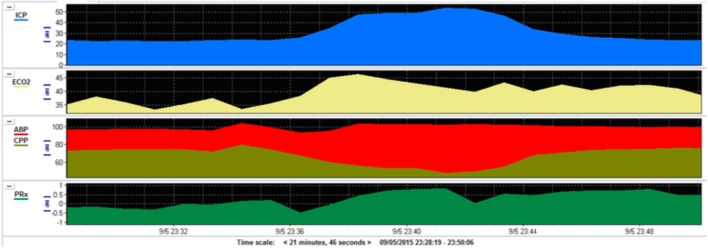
Multimodal monitoring during plateau waves in intracranial pressure (ICP) after traumatic brain injury (TBI). This is a pediatric patient who sustained severe TBI with moderate diffuse axonal injury (Marshall grade 3) on initial computed tomography scan. During the monitoring on the pediatric intensive care unit the patient developed rapid and short-lived increases in ICP (blue) in a pattern known as intracranial plateau waves. This corresponds with a reduction in cerebral perfusion pressure (CPP, gold) and a deranged cerebrovascular autoregulation (PRx, green). PRx—pressure reactivity index—is a measure of the capacity of the cerebral vasculature to alter its resistance in response to changes in CPP. A negative PRx indicates intact pressure reactivity whereas a positive PRx indicates impaired pressure reactivity.
